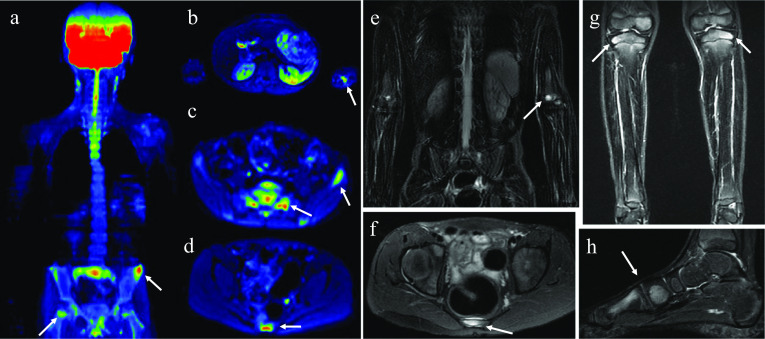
6-year-old male child with history of pain in his right hip and alteration of his gait for 6 months, with positive inflammatory tests, without trauma or fever and an investigation of hip synovitis. Initial MRI of the pelvis showed periphyseal lesions with symmetrical and bilateral distribution, in addition to involvement of the lower lumbar spine (not shown), raising the possibility of CNO. The WB-MRI study was suggested and performed 24 h after the first examination (a–h). DWI sequences (a–d) showing lesions already identified in the previous pelvic examination and detection of a new asymptomatic lesion in the capitellum (b), also demonstrated by the morphological sequence STIR (e). STIR sequences of the pelvis (f), legs (g) and left foot (h), with peripheral edema in the vertebrae, lateral tibial plateaus and in the medial cuneiform region and in the first metatarsal region, respectively. In WB-MRI, some lesions were detected in asymptomatic sites, demonstrating the multifocality and characteristic distribution of the disease and confirming its diagnosis
